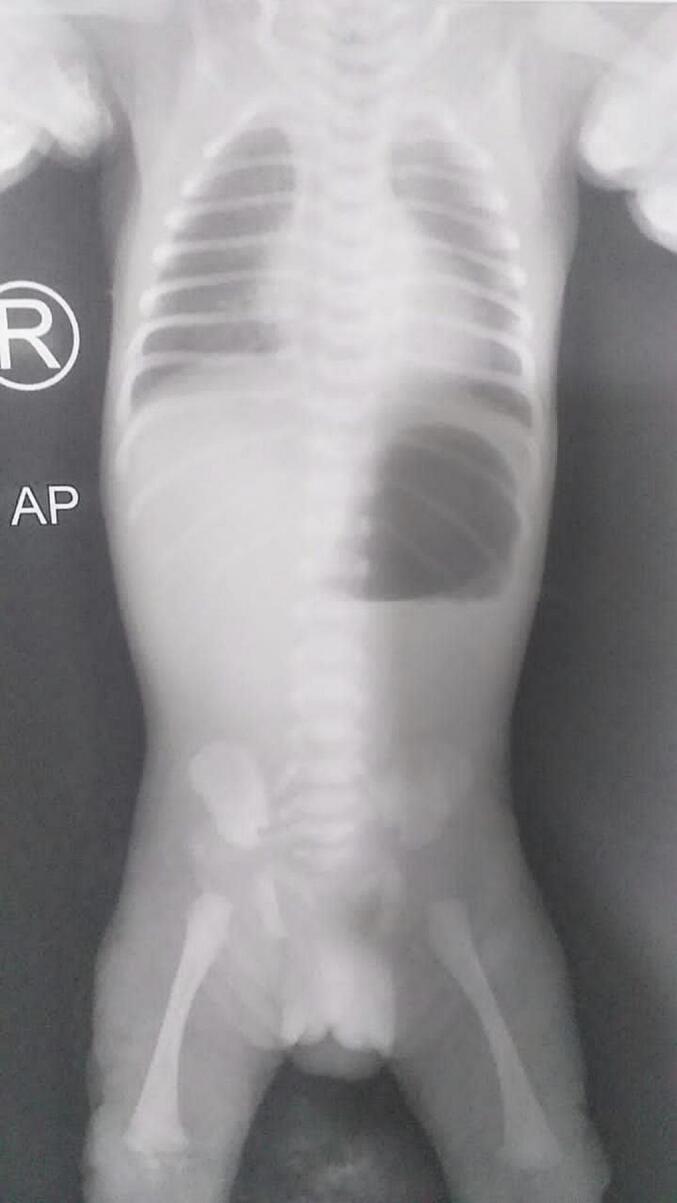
A plain abdominal X-ray demonstrated a huge, dilated stomach.
